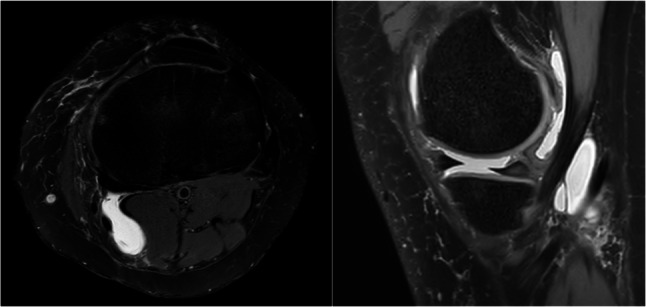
Sagittal and axial T2-weighted magnetic resonance images demonstrating a Baker’s cyst in the gastrocnemius–semimembranosus recess
